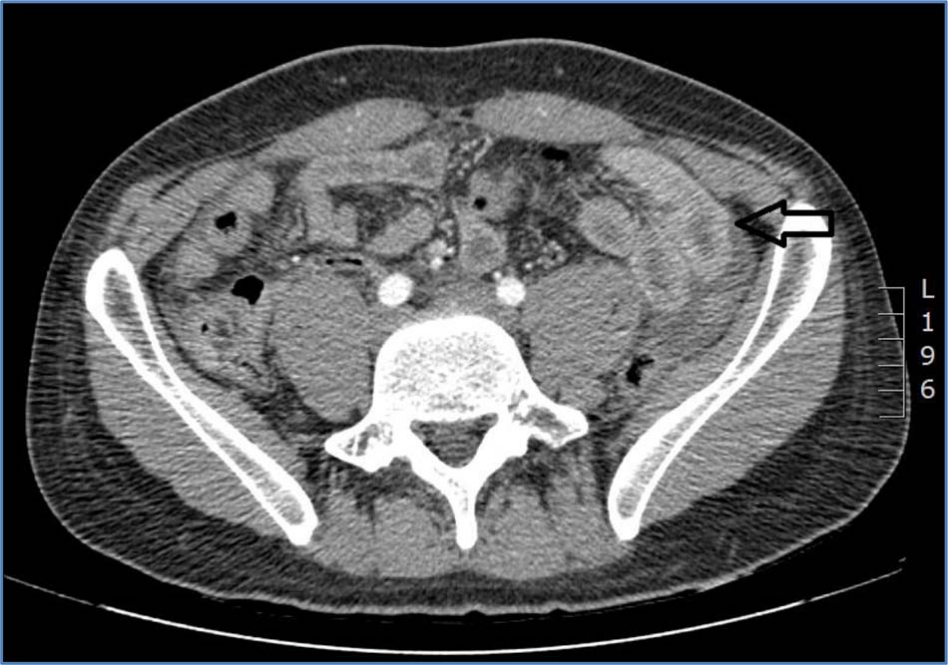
Abdominal CT scan showing distention, bundling and thickening of some small bowel loops in the left quadrant (arrow).
